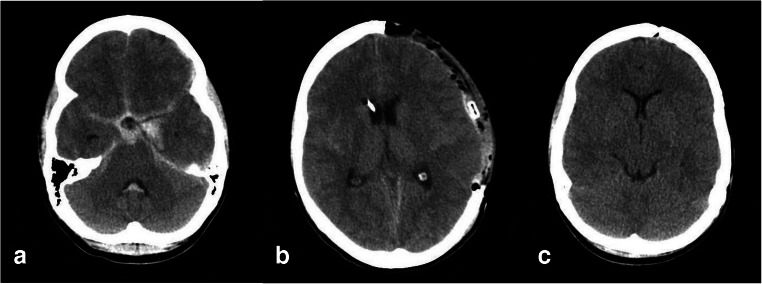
CT scans of a 21-year-old WFNS grade 5 aSAH-patient: preoperative scan (a), 24 h-scan after clipping and PDC (b), and after cranioplasty 3 months later (c)
